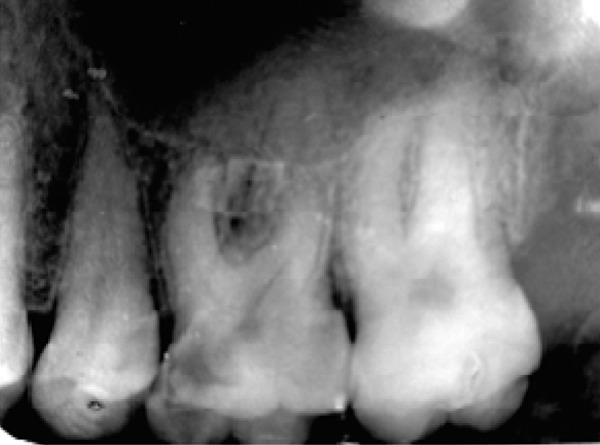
The initial radiograph of tooth #26
